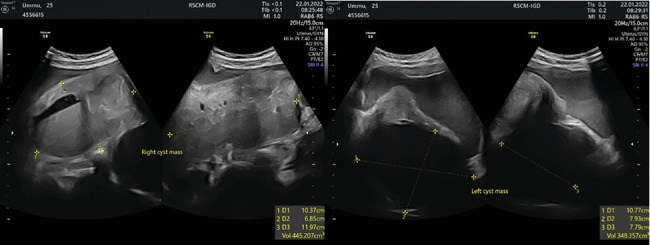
Ultrasonographic examination on right and left ovaries showing cystic mass.
